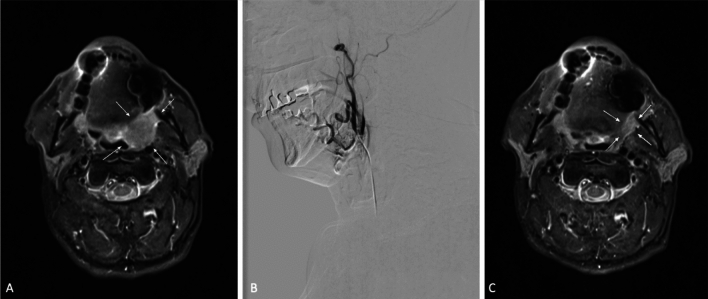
The case of a 61 year-old male with oropharyngeal squamous cell carcinoma is presented. Image A shows the MRI scan of the tumor prior to therapy. Image B displays the selective catheterization of the tumor-feeding branches of the external carotid artery. A combination of mitomycin c, gemcitabin, and cisplatin was administered. Image C shows the MRI scan of the tumor after multiple TACP sessions. The arrows point to the tumor. Overall, a PR was achieved, with a 60.46% reduction in tumor volume
